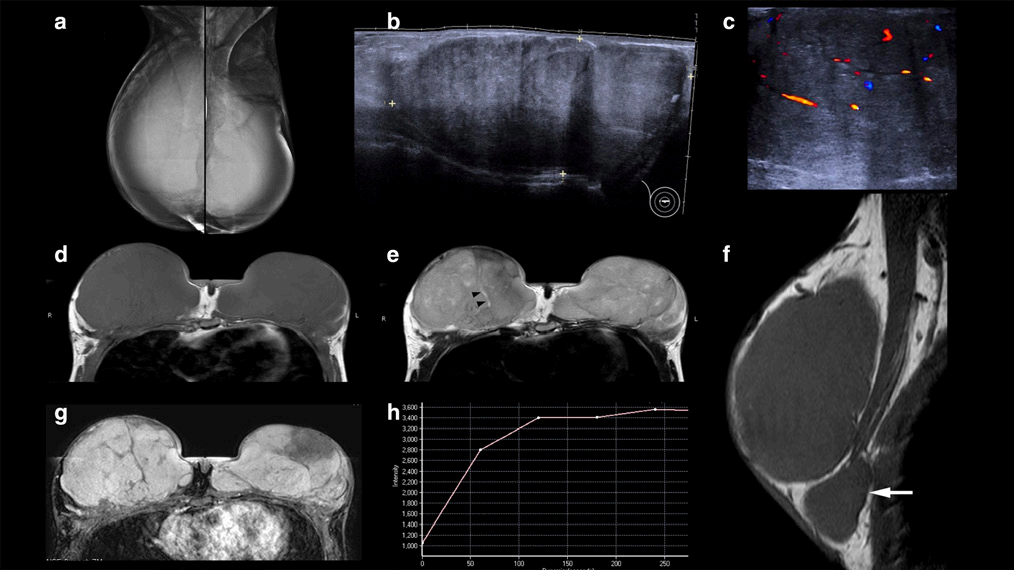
(A-H) A 13-year-old girl with bilateral PASH. (A)The mammogram reveals extremely dense breasts, due to a large equal-density mass in each breast. (B) Panoramic view ultrasonography of the right breast shows a large, oval, circumscribed, heterogeneous hypoechoic mass, measuring 13 cm in the longest diameter. (C) Doppler ultrasonography shows increased internal vascularity of the mass. On MRI, there is a large oval circumscribed iso-intensity mass occupying each entire breast on (D) axial *T_1_
